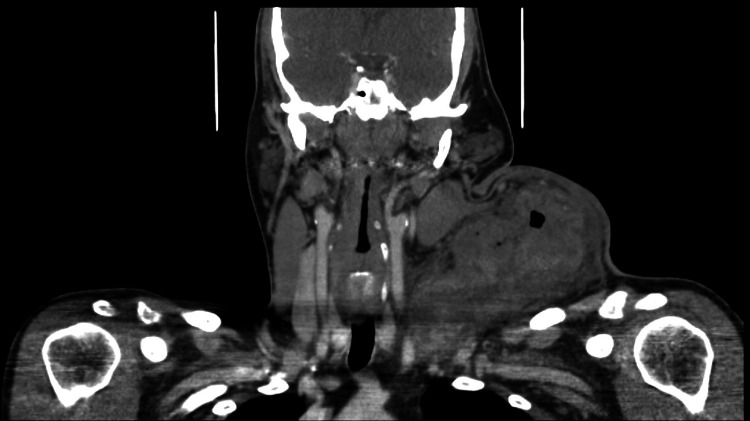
Coronal image of soft tissue neck obtained using CT scan with contrast revealed that there was a large, complex, ulcerated mass in the left neck measuring approximately 12 x 10 x 15 cm. The median margin abuts the carotid sheath and there is attenuation of the internal jugular vein. The inferior margin approaches the thoracic inlet including the great vessels. This has the appearance of a necrotic, ulcerated malignancy with associated inflammation. CT: Computed tomography
